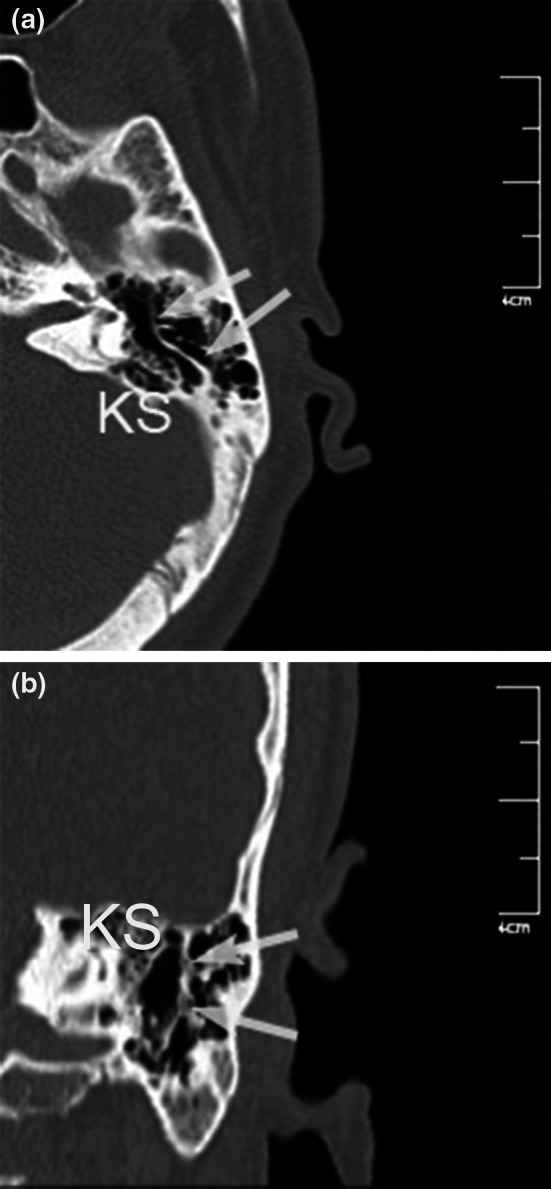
Reformatted computed tomography images. a Oblique axial image. b Coronal image. The Körner (petrosquamosal) septum (*KS*) is noted
